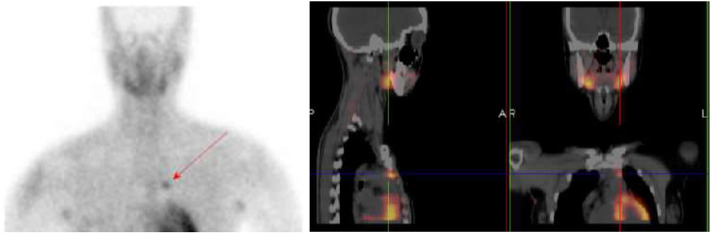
Sesta-MIBI parathyroid scan combined with SPECT-CT study of the neck-mediastinum showing the anterior mediastinal parathyroid adenoma.
